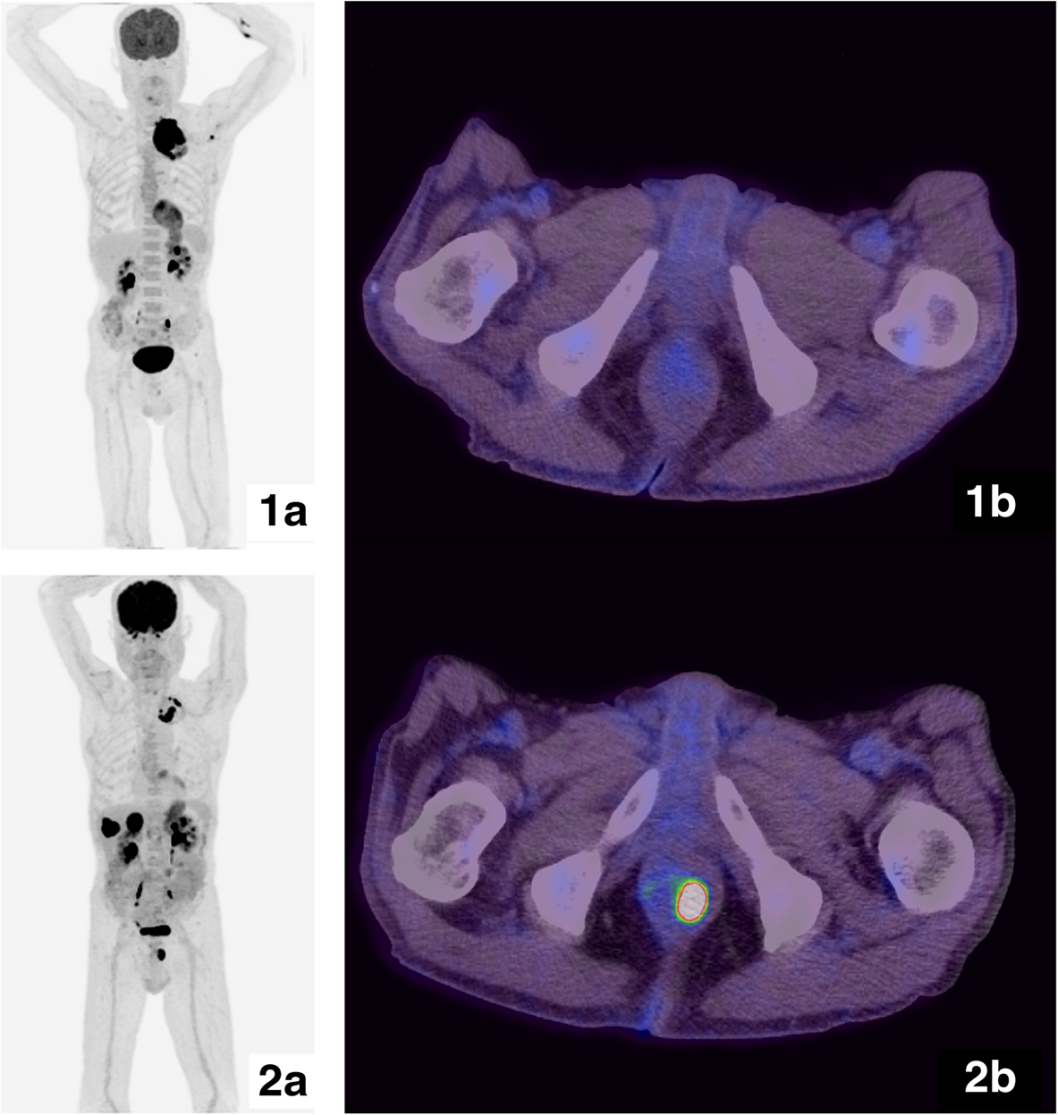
Maximum intensity projection (MIP) image of ^18^F-fluorodeoxyglucose-staging PET/CT showing hypermetabolic left-lung primary and mediastinal/left axillary lymph node involvement (1a). Transaxial superimposed PET/CT images showing no focal pathological uptake at the anus (1b). After four cycles of chemotherapy, the MIP image revealed new involvement of the adrenals, multiple bone metastases, and perianal uptake. Partial metabolic response in the left primary lung tumor (2a). Transaxial superimposed PET/CT images showing intense focal uptake at the left aspect of the anus (2b)
